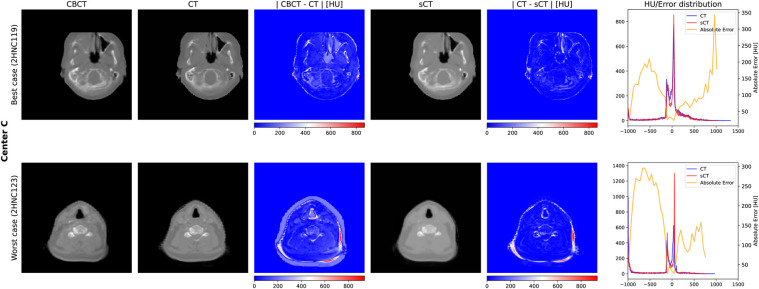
Visual comparison of the best and worst sCT cases from Center C. Compared to the other centers, Center C showed fewer registration-related errors and better image similarity, as evidenced by both the error maps (|CT−sCT|) and the distribution plots. However, case 2HNC123, identified as the worst-performing example, exhibited evident misalignments prior to the sCT generation despite the additional registration process.
